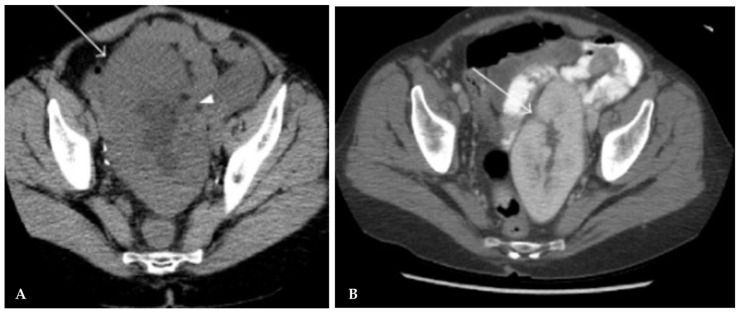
Non-contrast (A) and contrast enhanced (B) CT scan of the abdomen and pelvis. (A) Enlarged left lower quadrant renal allograft (arrow) with hilum directed laterally (arrowhead). (B) Previous imaging with an anteromedially directed hilum (arrow). Image reused from “Renal Allograft Torsion: US and CT Imaging Findings of a Rare Posttransplant Complication”, by Dewan S et al., published by Case Reports in Radiology, accessed on 18 December 2025, licensed under CC BY 4.0 (http://creativecommons.org/licenses/by/4.0/) [64]. Minor modifications were made to the title and descriptive captions. The background and size of the figure were also adjusted from the original source.
